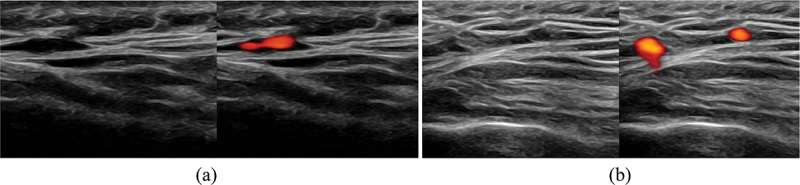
Normal ultrasound with colour Doppler of right superficial temporal artery without perivascular oedema or thickening; longitudinal (a) and cross-sectional (b) view.
